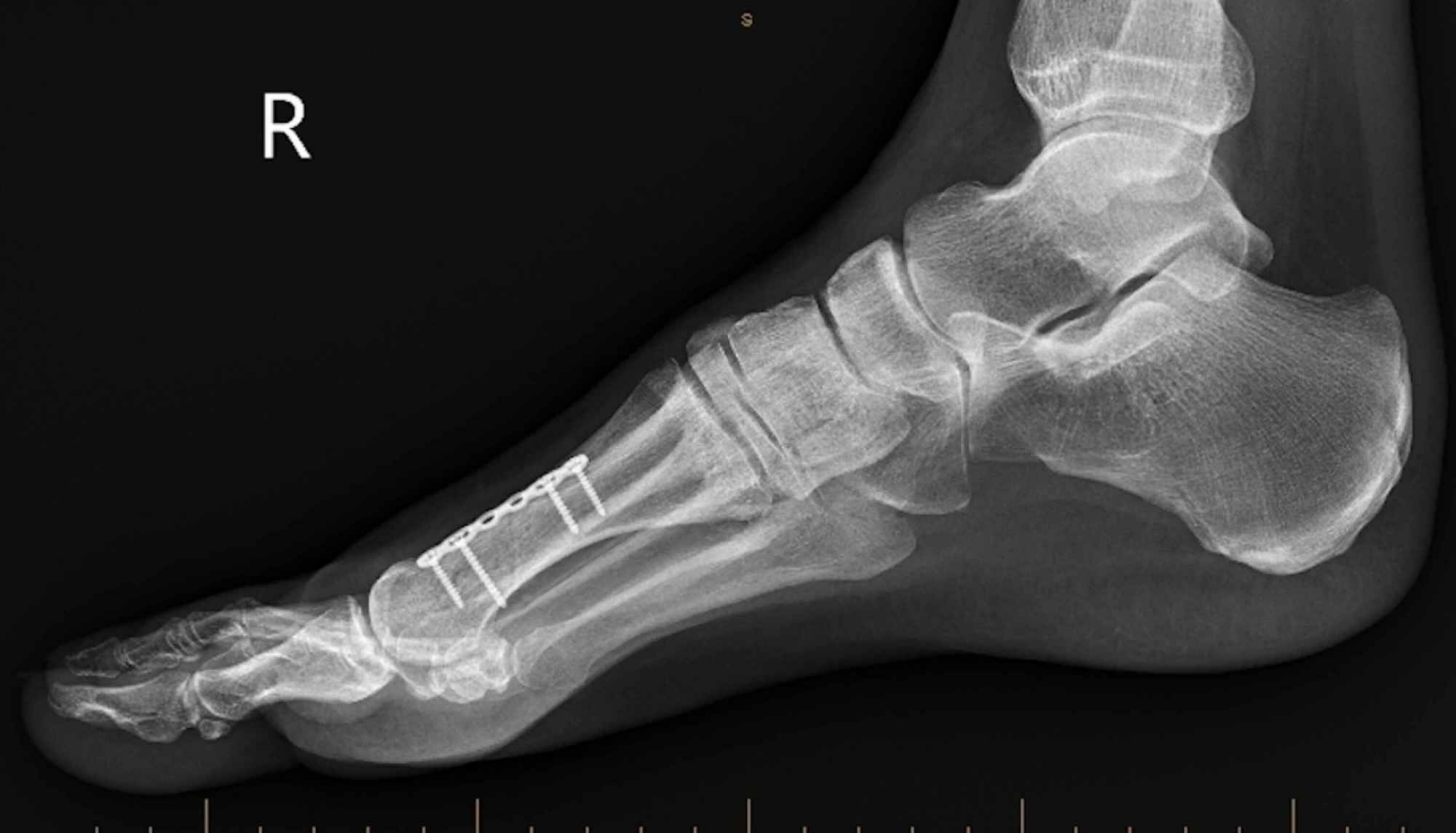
Post-operative lateral radiographs showing the return of the second metatarsal to its correct anatomical position
